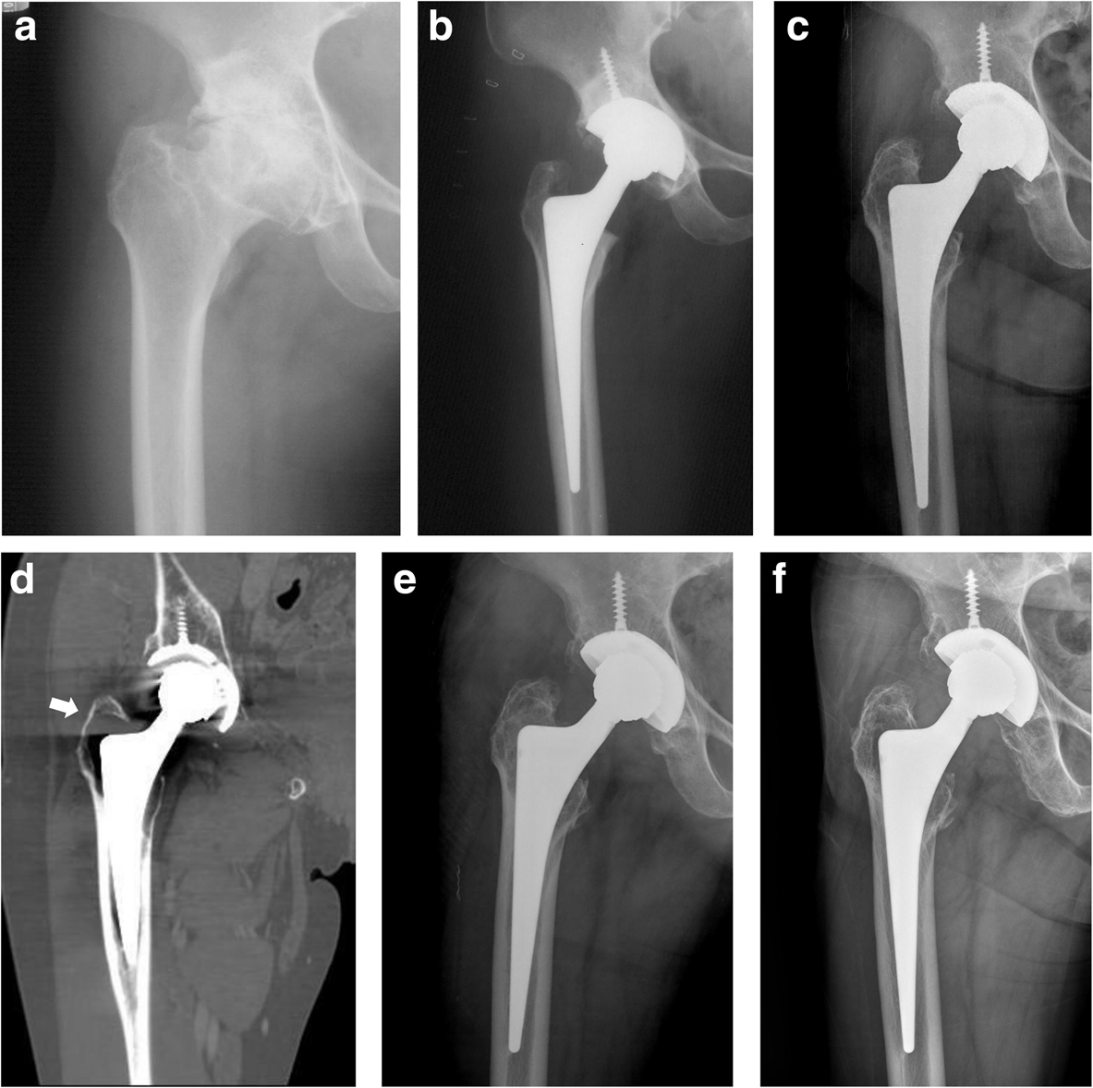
Osteolysis around the femoral stem. a Preoperative anteroposterior radiograph of the right hip showing osteoarthritis. b Immediate postoperative anteroposterior radiograph showing a well-fixated metal-on-metal articulated total hip arthroplasty. c–d An anteroposterior radiograph of the right hip at 11 years after operation. Computed tomography (coronal view) image before revision, showing an osteolysis around the proximal femoral stem, especially the greater trochanter (white arrow). e One year after revision with bone grafting around the greater trochanter. f An anteroposterior radiograph of the right hip at 21 years after total hip arthroplasty
